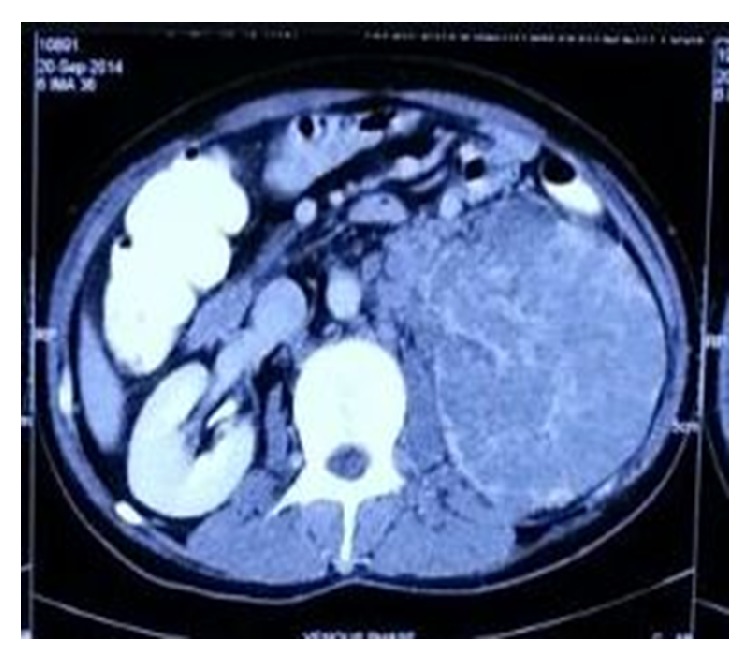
CT image shows enlarged left kidney and it is almost completely replaced with heterogeneously hypodense material.
